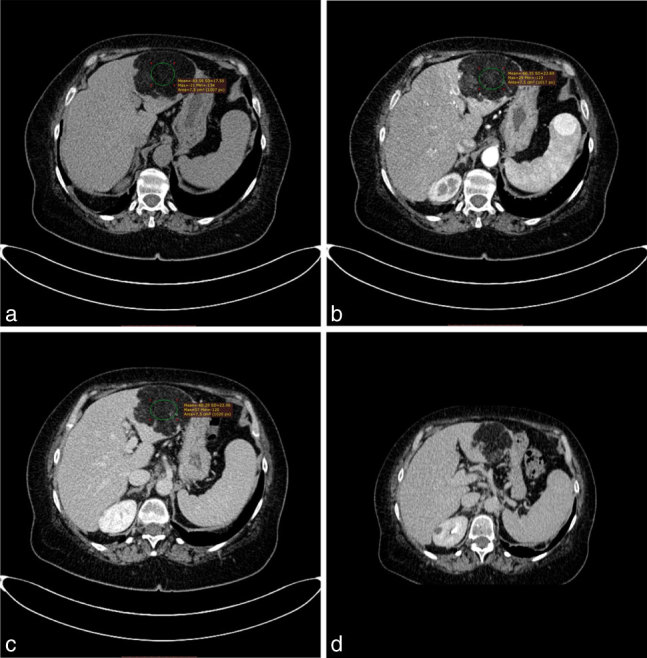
CT examination with contrast medium. (a) TC baseline, (b) arterial phase; (c) portal phase; (d) delayed stage coronal acquisition with axial reconstruction. It could be noted that the lesion has a similar attenuation of fat and does not show significant enhancement in subsequent phases of the study. Please note the presence of a splenic angioma. A region of interest (green ring in the figures), was placed on lesion and it shows the HU attenuation changes in different phases of the CT examination. The HU values do not change significantly in the different phases of acquisition. HU, Hounsfield.
